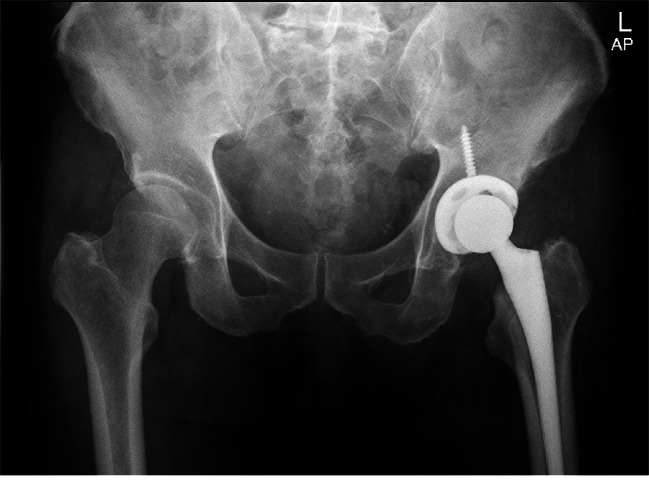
A standard postoperative AP pelvis X-ray
